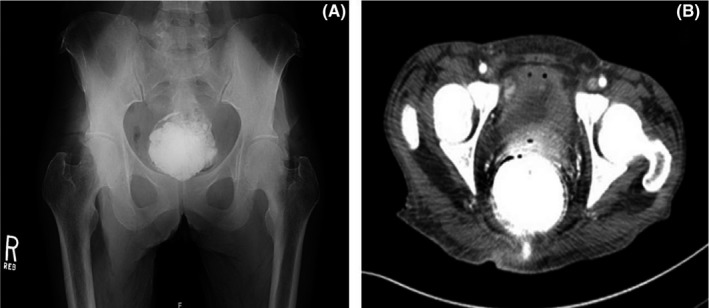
A, the abdominal x‐ray showed a large radiopaque abnormality, measuring about 7.5 × 6.3 cm, suggesting the possibility of retained barium. B, CT abdomen and pelvis with oral and intravenous contrast were consistent with barolith fecal impaction with evidence of stercoral colitis
